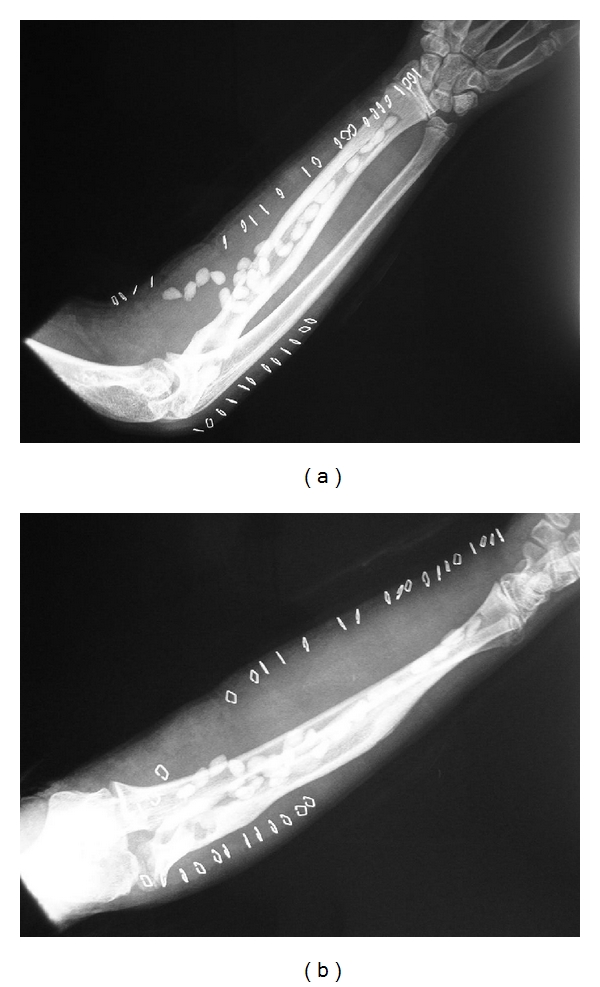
Postoperative AP and lateral radiographs of forearm.
